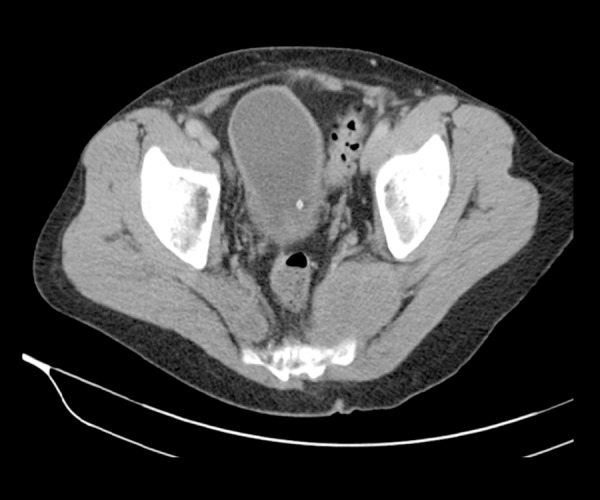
Image of the biopsied mass on CT in axial plane.
